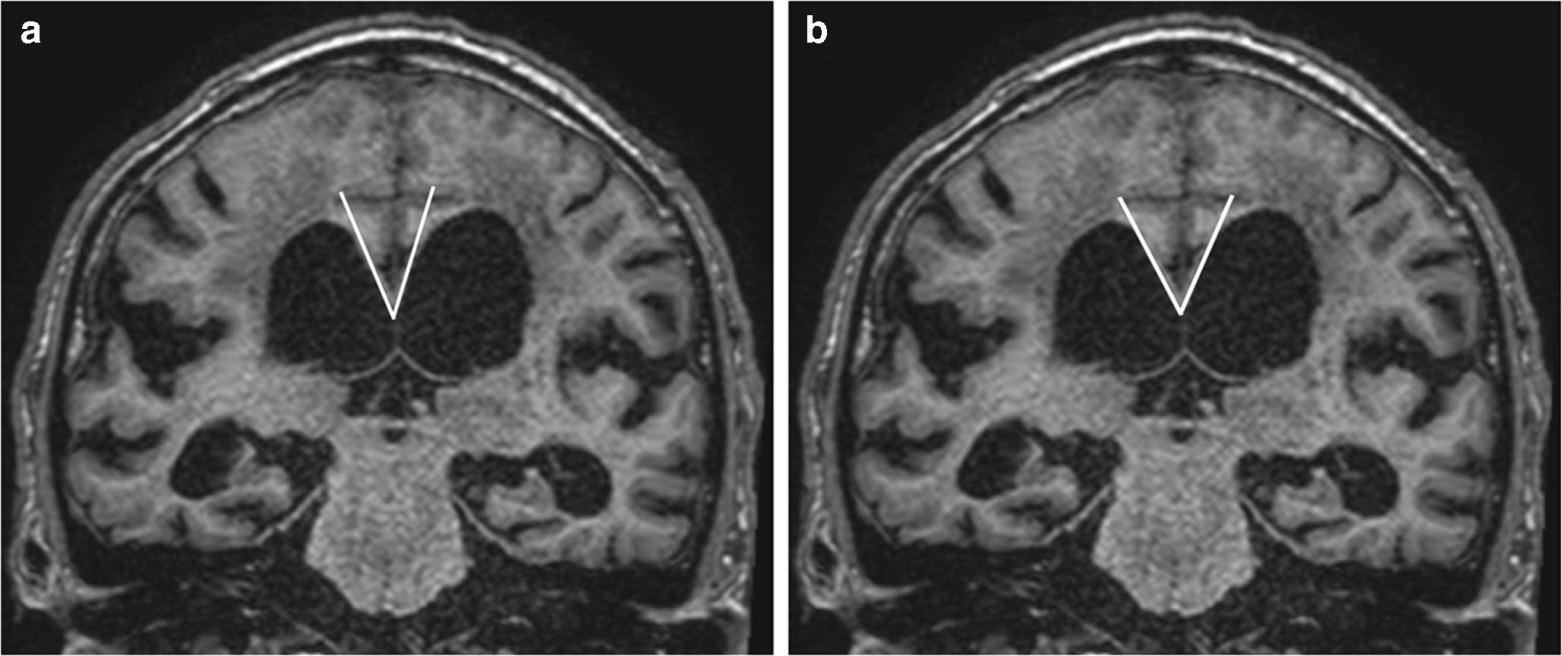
Coronal T1-weighted MR images show the callosal angle measured in the same coronal section with tangents in different positions along the curvature of the ventricular roof
